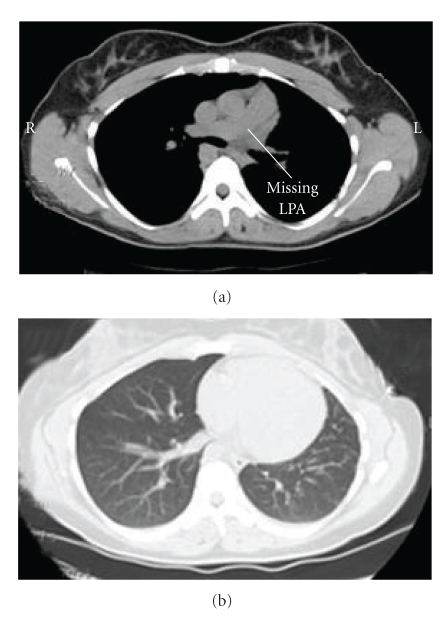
Noncontrasted chest CT shows a small left lung with left pulmonary artery aplasia. The right lung was normal.
